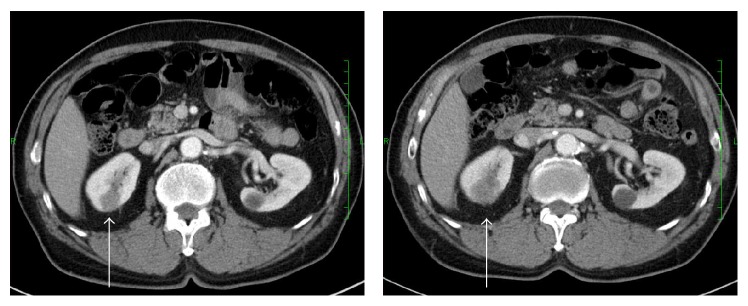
Computed tomogram of the abdomen showing right renal metastasis (arrow). The tumor increased in size during follow-up.
